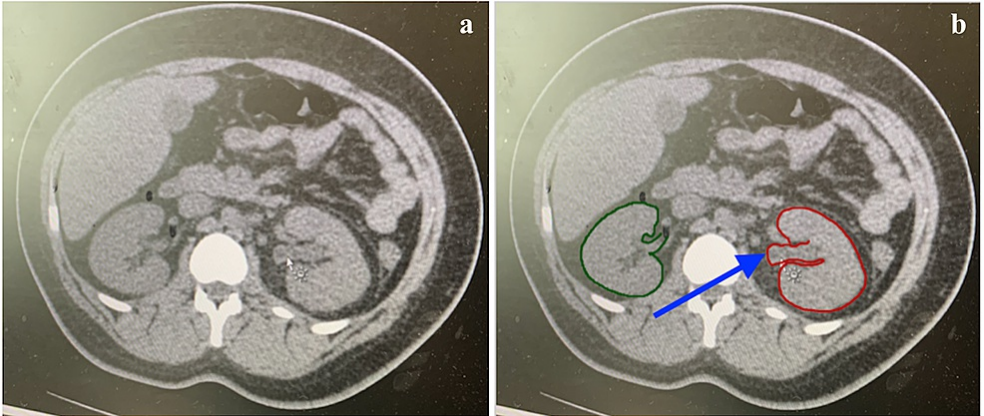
Non-enhanced axial CT scan images of the abdomen and pelvis at presentation. (a, b) Moderately inflamed enlarged left kidney with perinephric edema. (b) Displays the enlarged left kidney by inflammation (red circle) and a dilated intrarenal collecting system (blue arrow).
